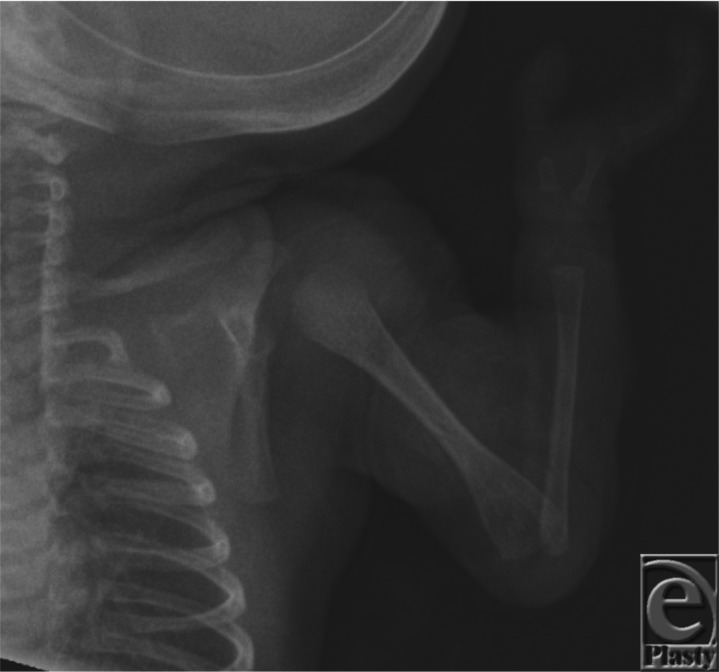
Radiograph showing absence of the ulna and ulnar three digits.
